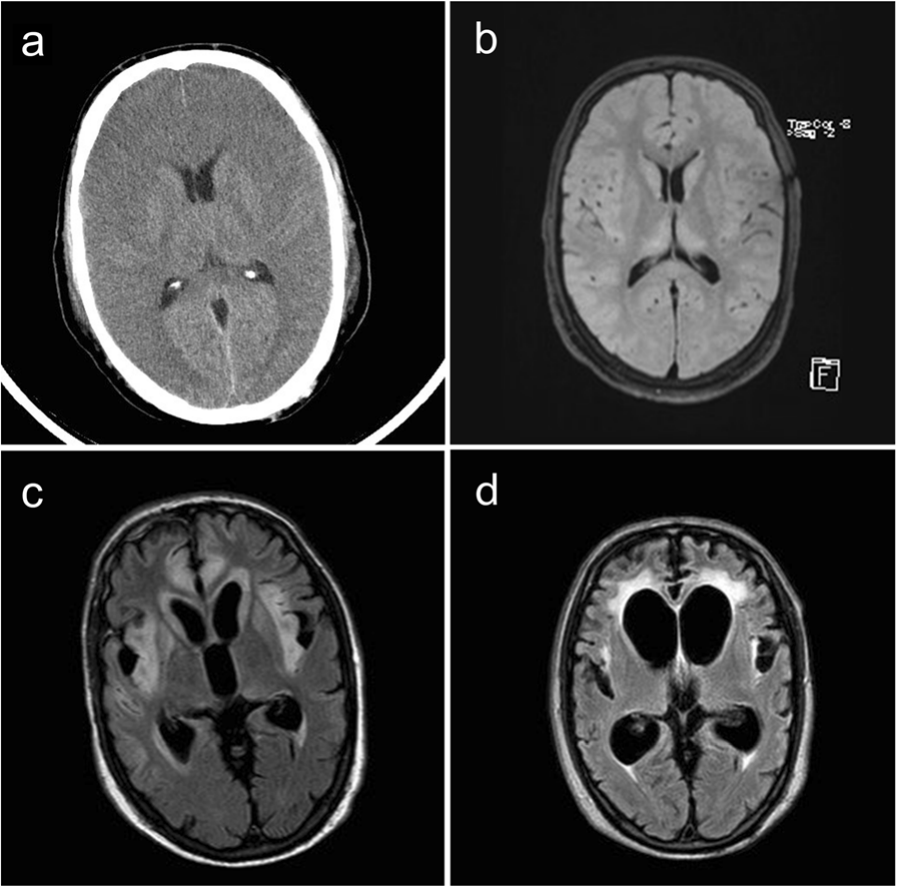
Cranial imaging at different time points from a 48-year-old man with acute hyperammonemia and hyperornithinemia-hyperammonemia-homocitrullinuria syndrome. Computed tomography of the brain 4 days after admission shows a considerable general cerebral edema (a). Magnetic resonance imaging at day 5 shows a widespread cytotoxic edema in insula, gyrus cingula, and the temporal and frontal lobes (b). Magnetic resonance imaging at day 31 demonstrates regression of edema, widespread gliosis in the frontotemporal lobes bilaterally, and hemorrhagic necrosis/malacia in frontotemporal cortex and basal ganglia bilaterally (c). Magnetic resonance imaging 7 months after admission shows pronounced progress of gliosis development, widespread malacia in the tips of the temporal lobes with greatly widened temporal horns of the lateral ventricles (d)
